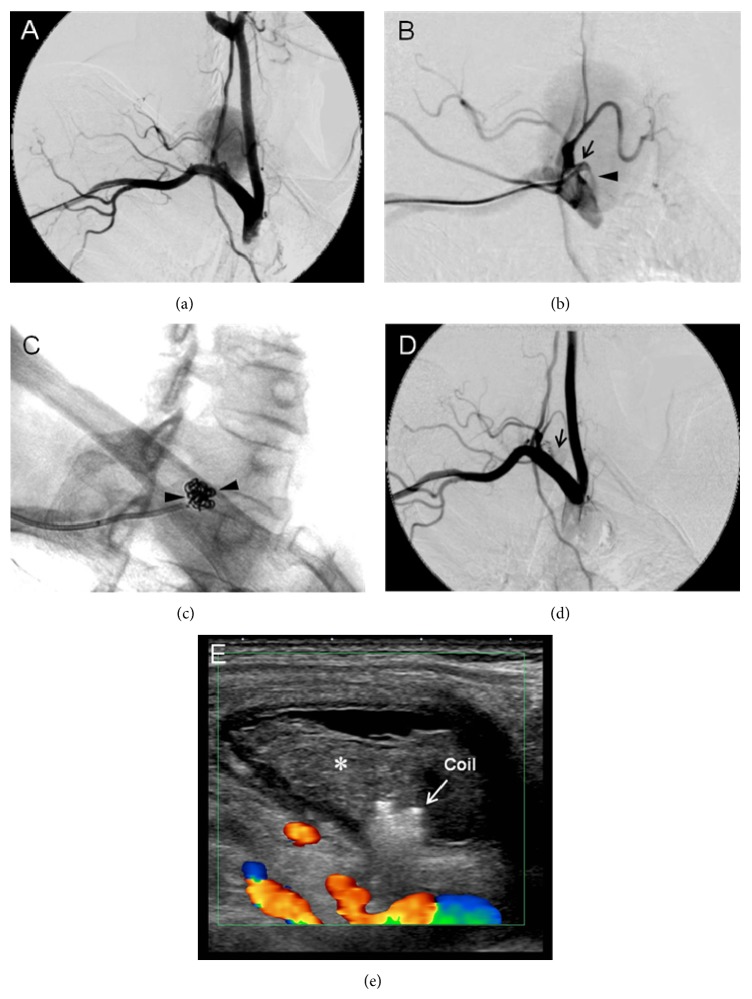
(a) Digital subtraction angiogram (DSA) in the right anterior oblique view showed a large PsA originating from the TCT. (b) Selective DSA of the PsA neck clearly showed the short narrow neck originating from the proximal side of the TCT root. (c) Coil embolization of the PsA. Arrowheads indicate the coils corresponding to the PsA neck. (d) DSA after coil embolization showed the near disappearance of the PsA, but faintly residual contrast dye was noted near the coil (arrowhead). (e) Duplex ultrasound following 15 min ultrasound-guided compression. Complete disappearance of blood flow within the PsA and thrombus formation (asterisk) was observed.
